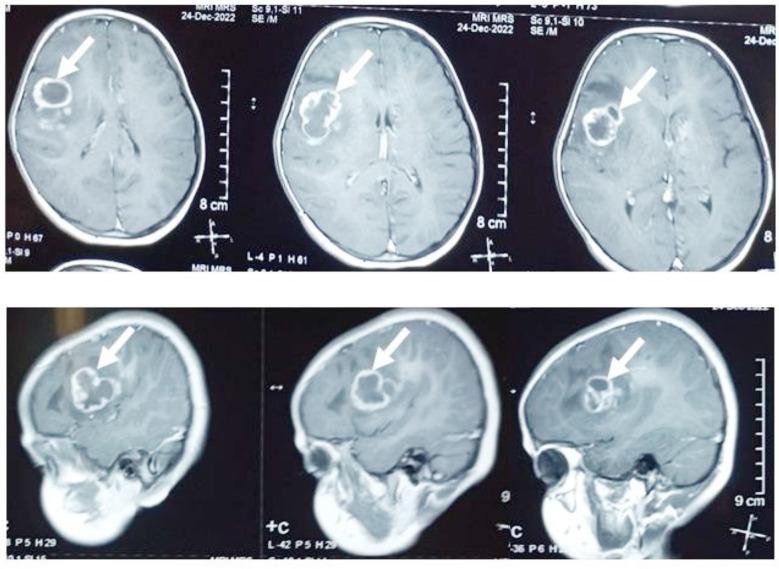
Case 1: MRI brain T1W post-contrast: Multiple marginally-enhancing and nodular lesions in right frontoparietal lobe with extensive peri-lesional vasogenic edema, ill-defined, heterogenous enhancement of left caudate nucleus head, and multifocal bilateral intra-axial lesion showing FLAIR and T2 hyperintensity.
